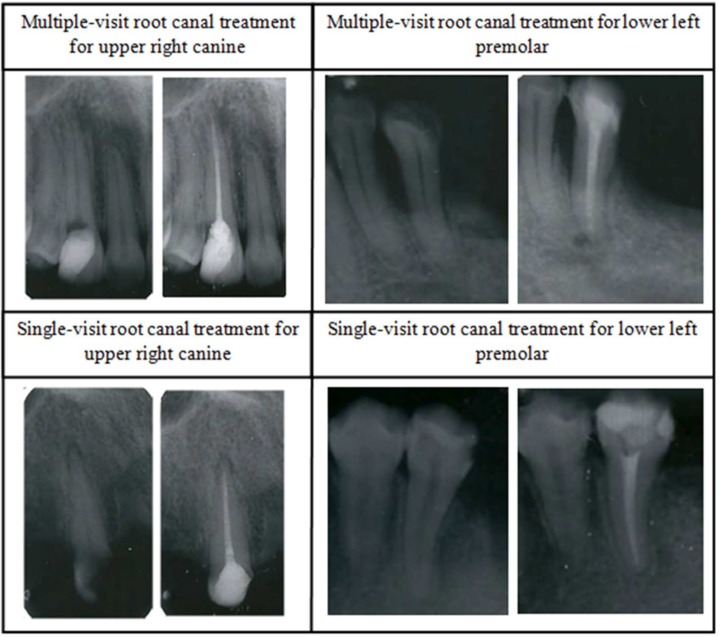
Example of matching of pre and postoperative periapical radiographs of anterior and posterior teeth treated in single and multiple-visit
